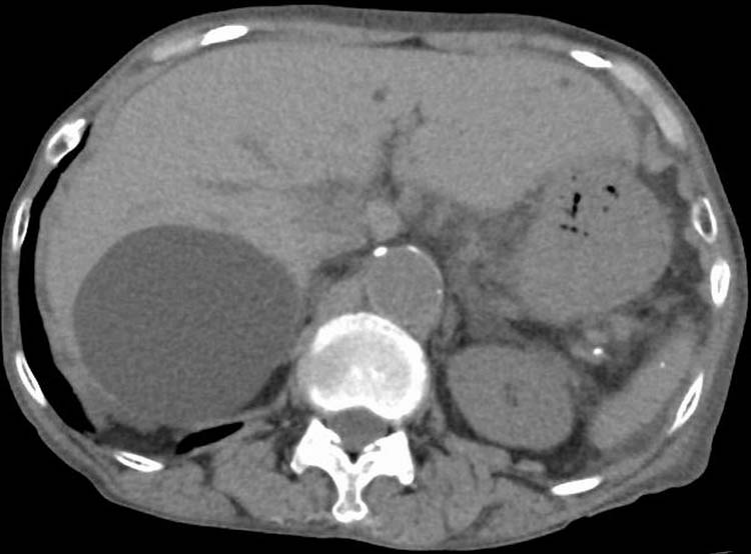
Abdominal CT 5 years prior to admission showing a cyst in the right hepatic lobe.
